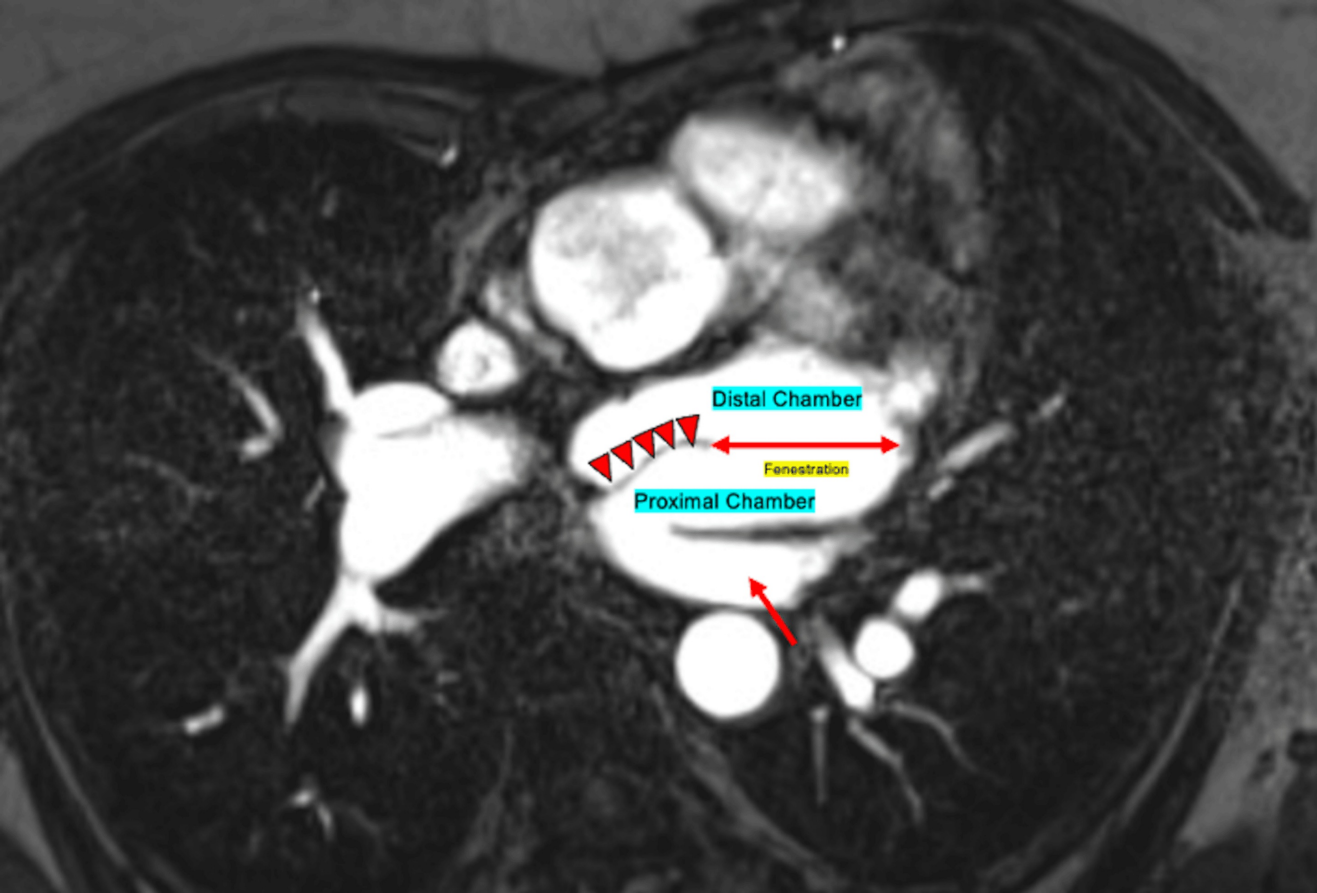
Cardiac MRI showing the LA divided into proximal and distal chambers by an abnormal septum (red arrowheads) The right pulmonary vein drains into the proximal chamber (red single-headed arrow). A fenestration is visible along the left lateral margin (red double-headed arrow). LA, left atrium
